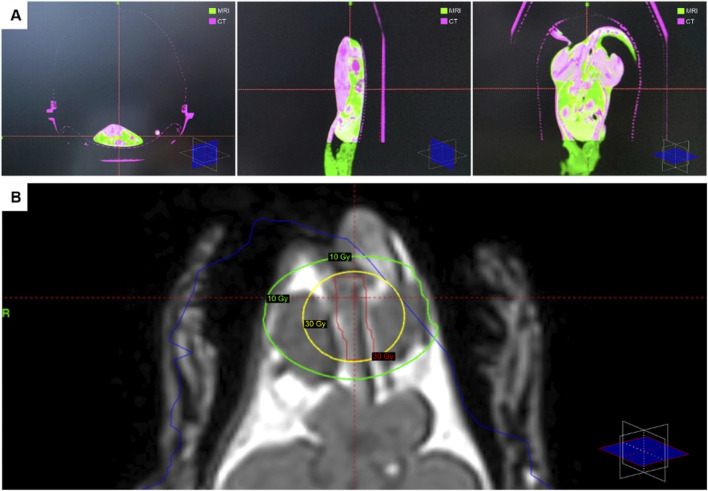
Individually customized stereotactic radiotherapy and dosimetric planning. (A) Stereotactic reference imaging in different scanning planes, using co-registered images from MRI and CBCT, automatically overlayed using skeletal landmarks. (B) Individually customized treatment plan, highlighting the anal canal (*outlined in red*), the dosimetric prescription (30Gy through the entire organ volume), the 30Gy beam area (*outlined in yellow*) and the surrounding 10Gy isodose (*outlined in green*).
